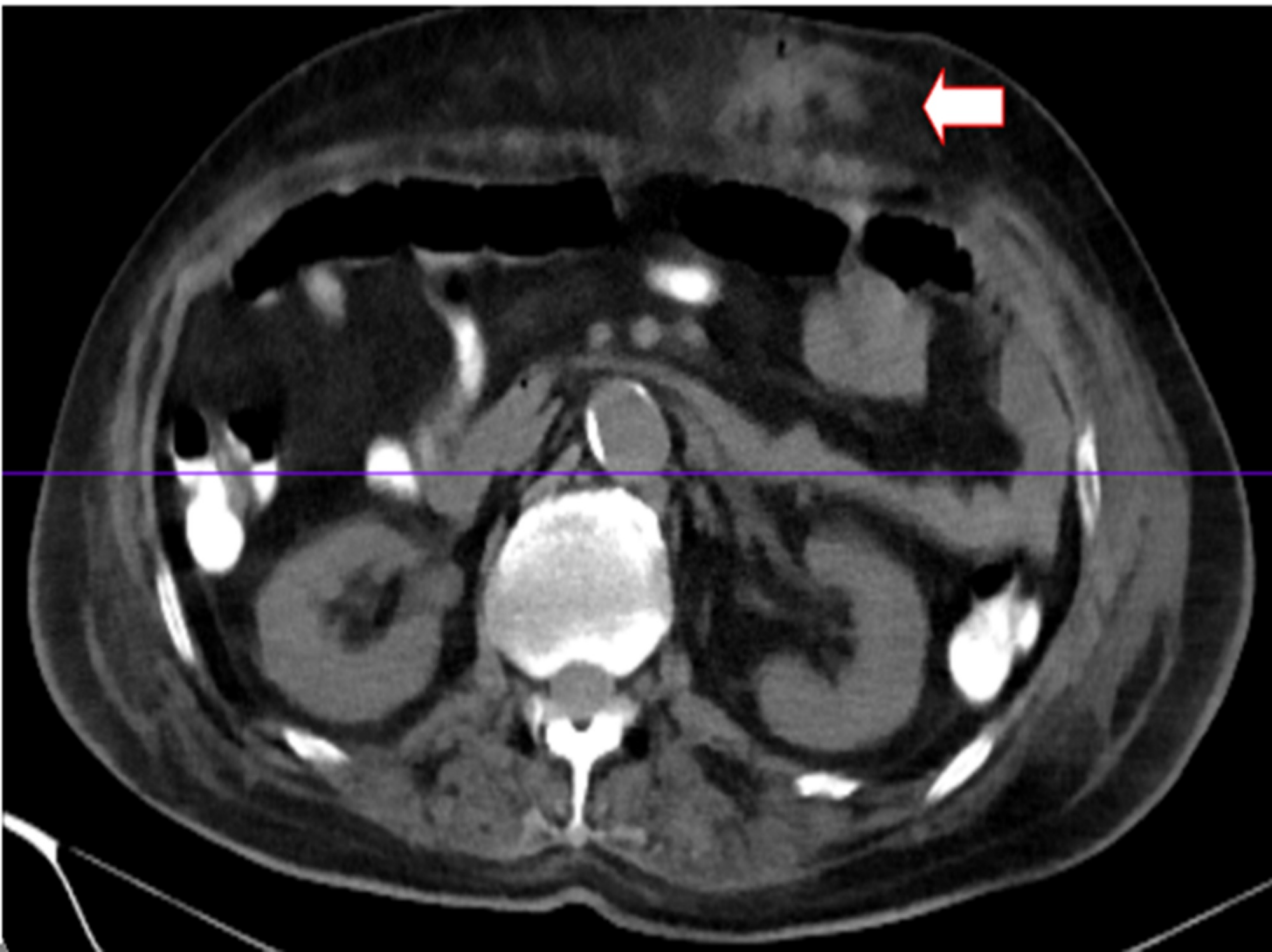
CT abdomen/pelvis: abdominal wall cellulitis with an abscess at PEG insertion site CT, computed tomography; PEG, percutaneous endoscopic gastrostomy
